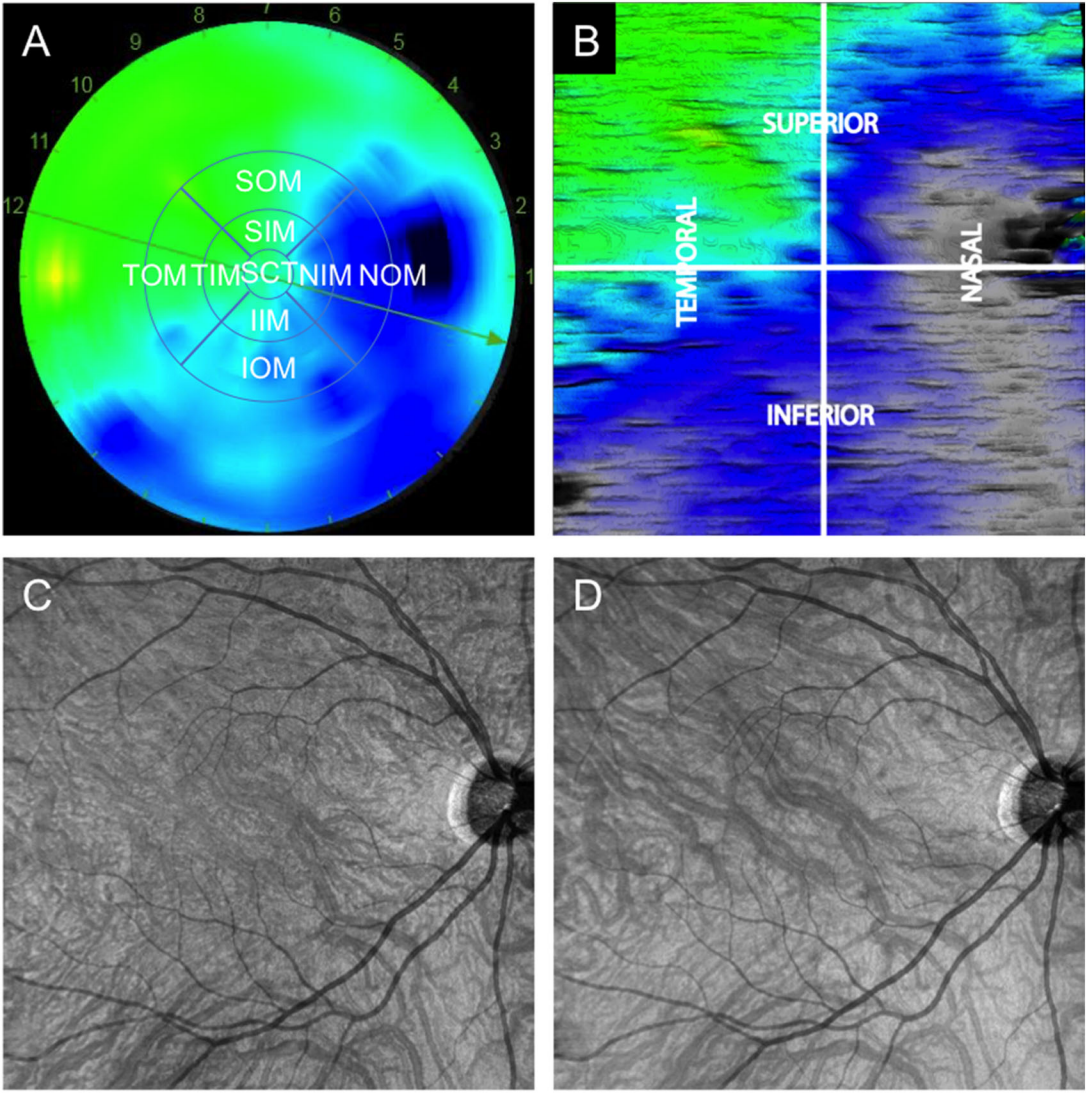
Example of choroidal thickness maps and corresponding en face OCT of the choroid. (A) Choroidal thickness (CT) map obtained from 12-mm radial B-scans provided an automatic measurement of the subfoveal CT (SCT) in the central 1-mm-diameter and the mean macular CT in nine subfields based on the ETDRS grid. The inner ring (3-mm radius) included the superior inner macula (SIM), temporal inner macula (TIM), inferior inner macula (IIM), and nasal inner macula (NIM); the outer ring (6-mm radius), the superior outer macula (SOM), temporal outer macula (TOM), inferior outer macula (IOM), and nasal outer macula (NOM). (B) Choroidal map obtained from the 9 × 9-mm OCT-A scan shows four quadrants centered on the fovea (superotemporal quadrant, superonasal quadrant, inferotemporal quadrant, and inferonasal quadrant) and allows measuring CT at any point of the map. The thickest choroidal point was determined on this map. (C) En face OCT images extracted from the 9 × 9-mm OCT-A scans showing choroidal vessel distribution at 100-µm depth from the retinal pigment epithelium. (D) To describe the course of choroidal vessels, we determined that a Bruch's membrane CSI slab covering the entire CT allowed the best visualization of the Haller's layer.
